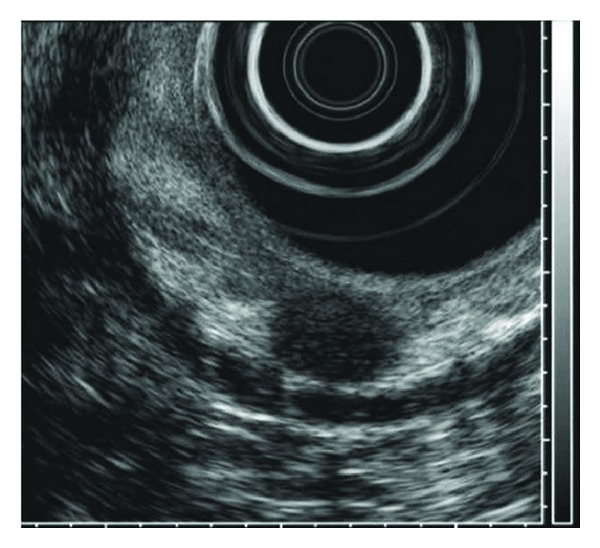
An approximately 1 cm × 0.8 cm, round, homogenous hypoechoic lesion located in the submucosal layer was detected using endoscopic ultrasonography.
